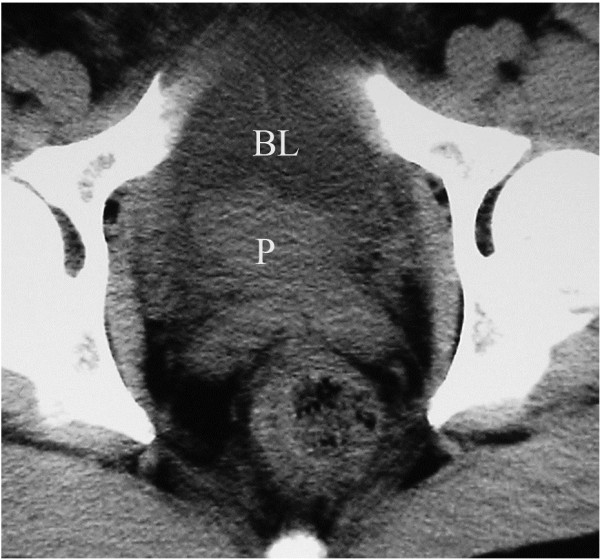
CT indicates mucosal thickening in the bladder wall and a shadow of soft tissue density in the bladder neck, which was considered to be a bladder-occupying lesion. P: prostate; BL: bladder.
